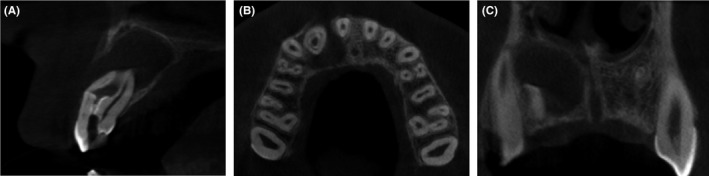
Preoperative cone‐beam computed tomographic (CBCT) presentation of the case. A, Saggital section. Note the large space of the invagination and the extremely compressed pulp space in the buccal side of the root and the presence of a large periapical radiolucency related to the tooth #12. Destruction had no connection with maxillary sinus or the nasal cavity but did penetrate the buccal wall. There was still viable a little of undamage bone structure around the real root canal space in the periapical area. B, Axial section. The invagination was separated from a circular real root canal and had a different portal of exit. C, Coronal section. The shape of radiolucency was quite oval, and it had a quite well circumscribed sclerotic border
